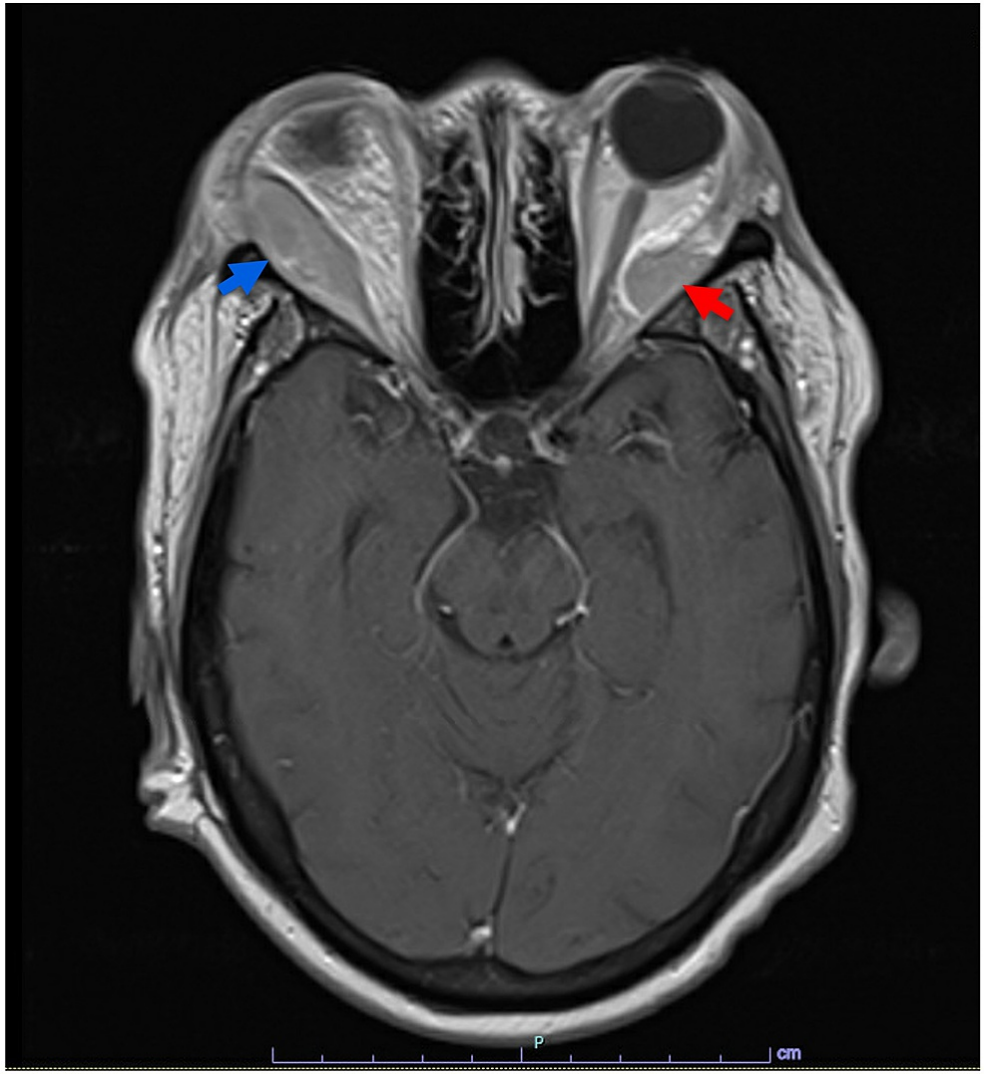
MRI brain on admission The right orbital mass (blue arrow) exerted a local mass effect on the lateral rectus muscle and the posterior lateral globe. The left orbital mass (red arrow) exerted a mild mass effect on the lateral rectus muscle MRI: magnetic resonance imaging
